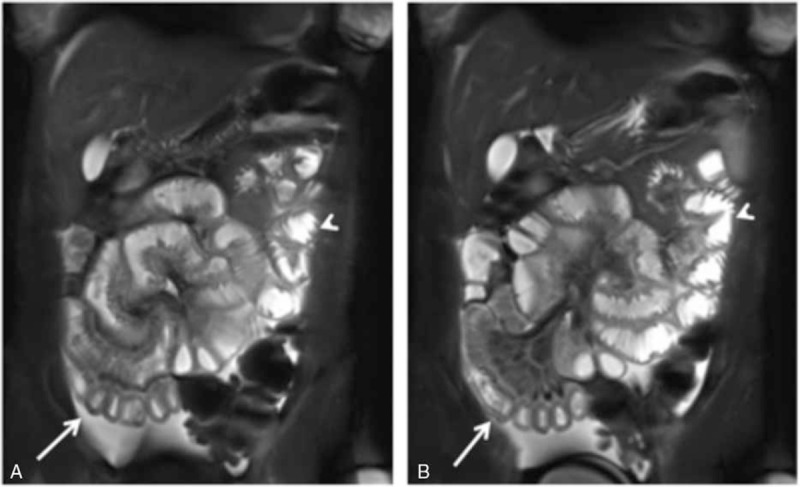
A, B: Abdomen MRI revealing dilated, fluid-filled small bowel loops and increased colonic wall diameters (arrows). MRI = magnetic resonance imaging.
